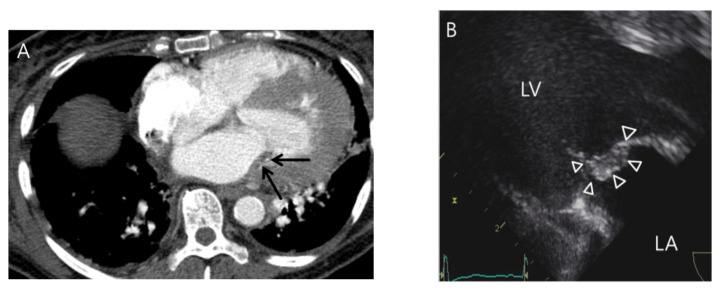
A case of elongated nodular thickening of the mitral valve without an indirect-oscillation sign demonstrated on routine chest CT. A 62-year-old woman presented with fever and dyspnea. (A) Elongated nodular thickening (arrows) at the lateral portion of the mitral valve was noted on an axial CT image. However, an indirect-oscillation sign was considered absent in this patient due to its adherent appearance to the mitral valve and the lack of a blind end. (B) The CT finding was confirmed to be a vegetation (arrowheads) with elongated appearance on subsequent transesophageal echocardiography.
